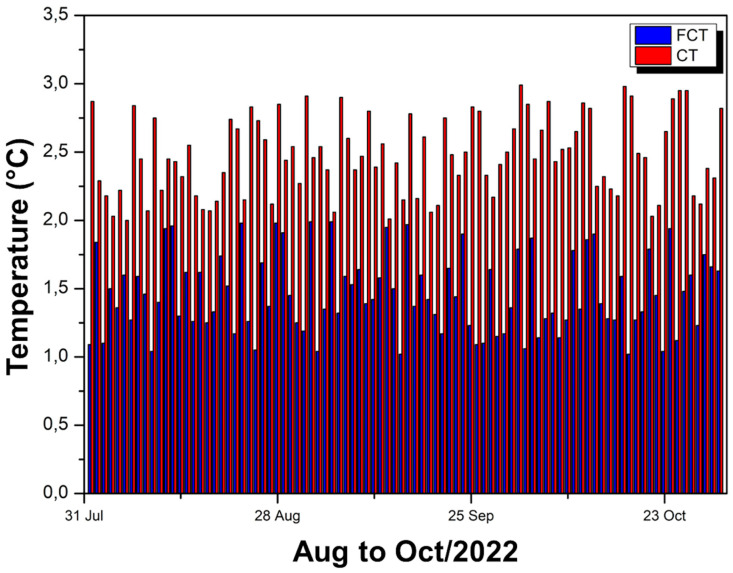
Comparison of internal temperatures of FCT and CT—Aug to Oct/22.
